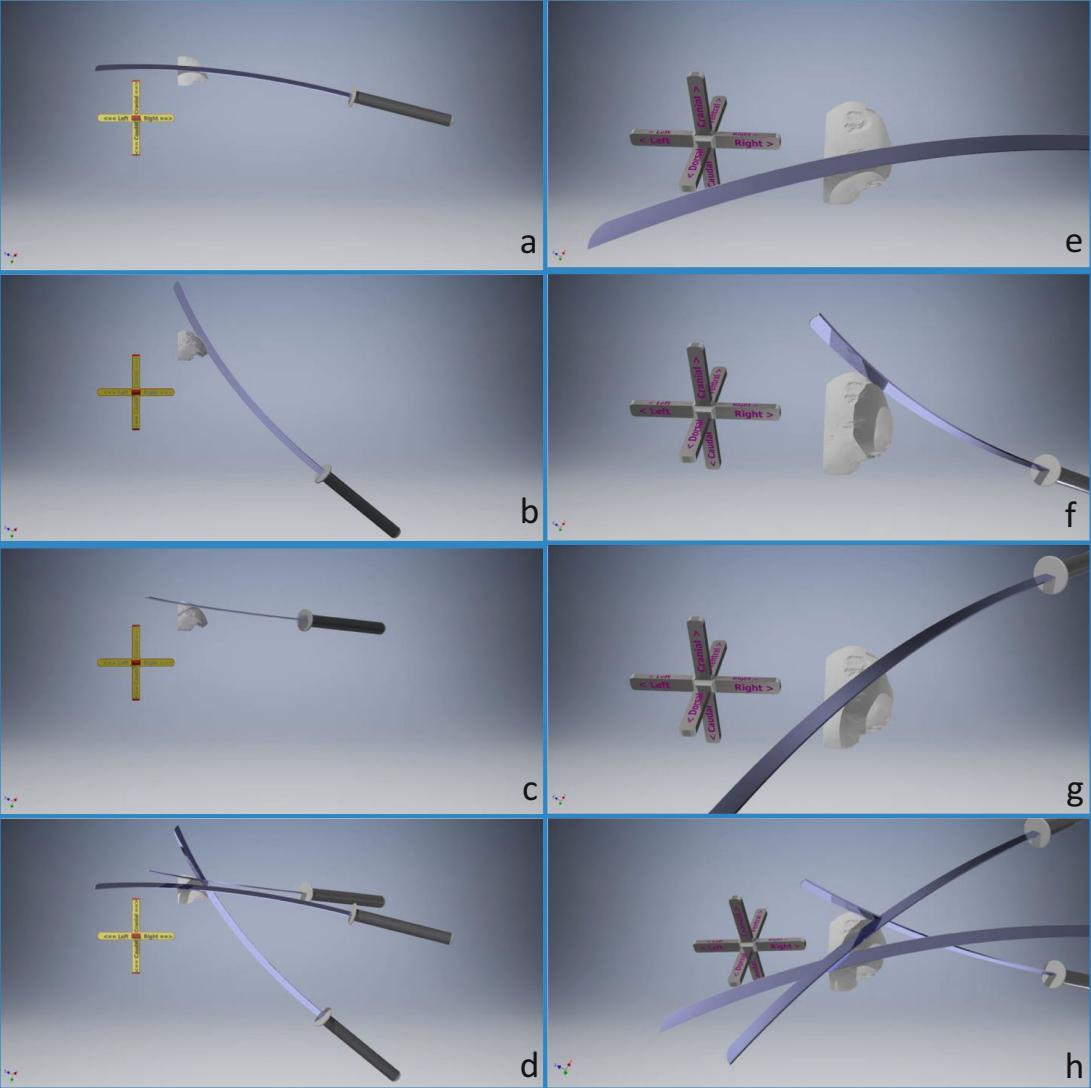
3D-CT model of the cranial bone section and CAD model of sword A. The sword is placed according to the results of the tool mark analysis. Panels a to d (posterior view) show the orientation of the sword when creating mark I (a), II (b), and III (c) and all together in d. Panels e to h show close-ups and from a different angle
